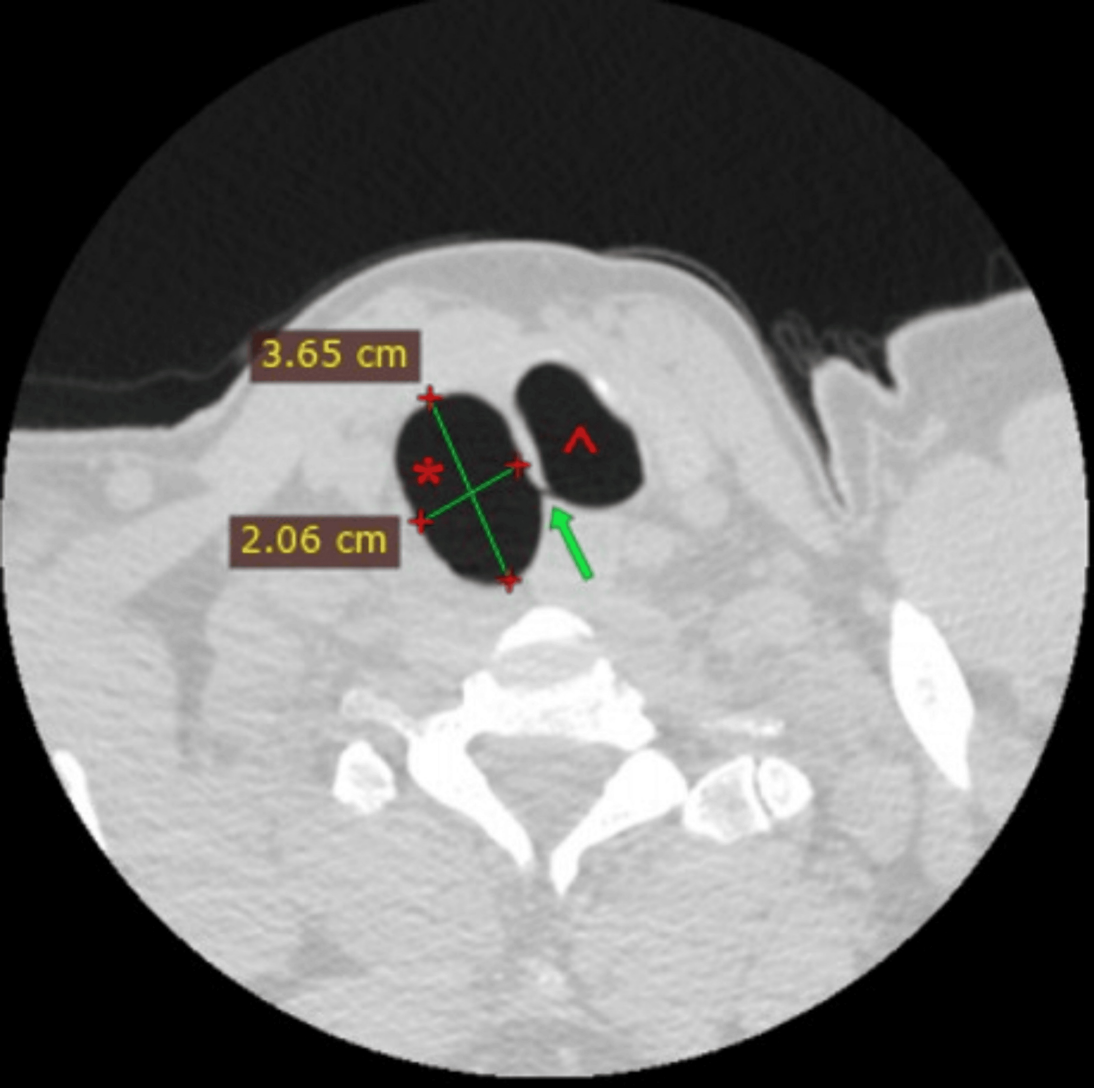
Second CT scan during Valsalva maneuver (1.25-mm slice), axial view Right-sided tracheal diverticulum (*) with dimensions of 3.65 x 2.06 cm, with detectable connection (green arrow) to the trachea (^)
